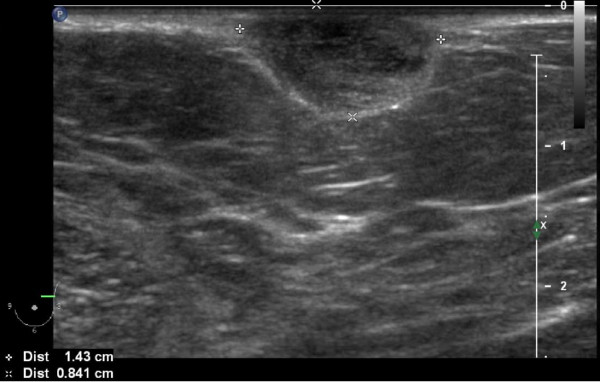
Ultrasonography. Nodular lesion in her left breast measuring 1.4 × 0.8 cm.
